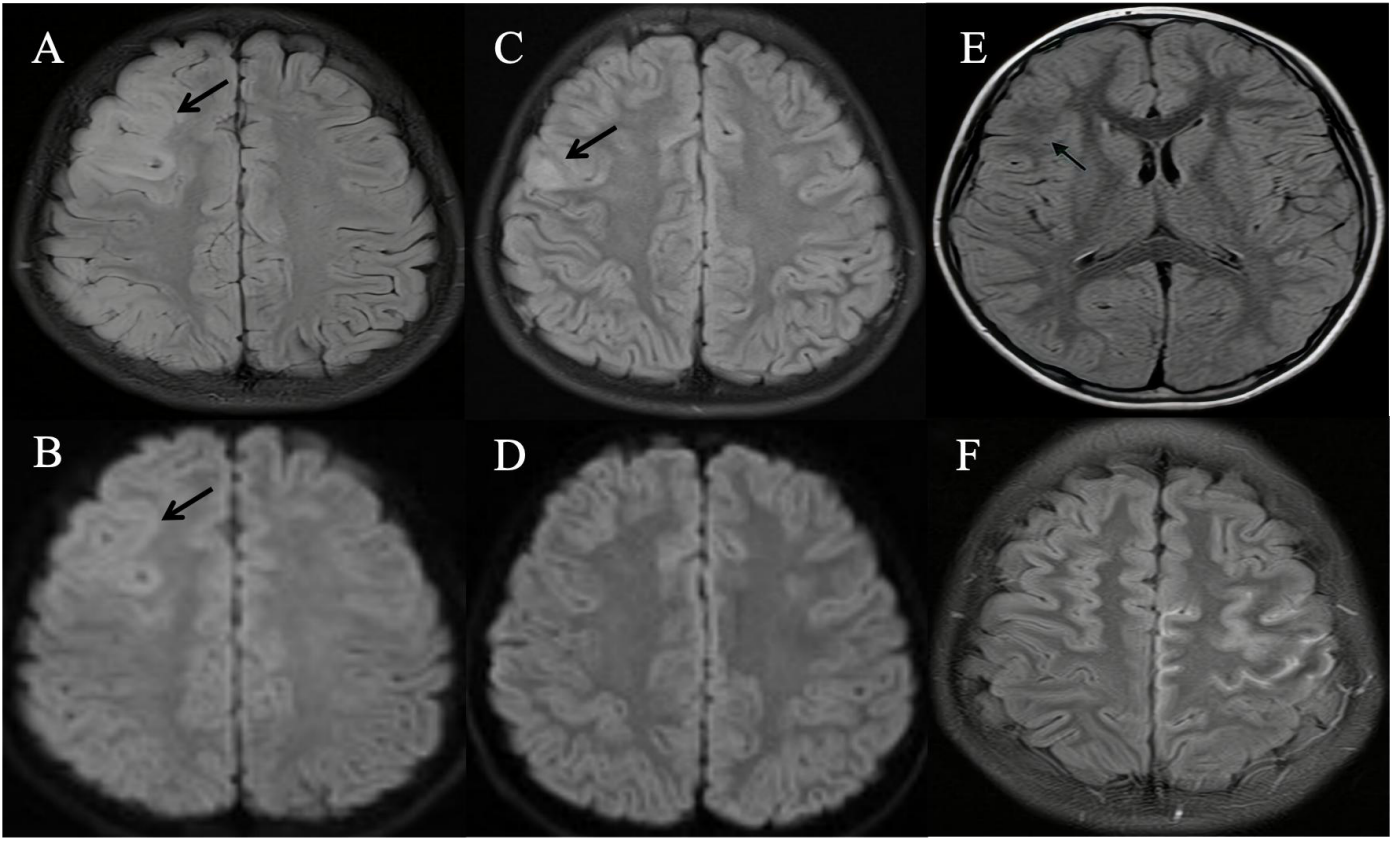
Brain magnetic resonance imaging (MRI) of patients with unilateral cortical FLAIR-hyperintense Lesion in Anti-MOG-associated Encephalitis with Seizures (FLAMES). (A) Fluid attenuation inversion recovery (FLAIR) image showed hyperintensity in the cortical region of the right frontal and parietal lobes, without white matter involvement (Patient 9). (B) Diffusion-weighted imaging (DWI) showed an obvious signal change in the cortex (Patient 9). (C) Hyperintensity in the cortical region of right frontal lobes in MRI FLAIR images (Patient 6). (D) DWI showed no obvious signal change in the cortex (Patient 6). (E) FLAIR image showed diminished signal intensity in the subcortical white matter at the lesion site in comparison to the contralateral hemisphere (Patient 3). (F) Gadolinium-enhanced T2-FLAIR imaging revealed multiple gyral enhancements in the left frontal and parietal cerebral cortex (Patient 5).
